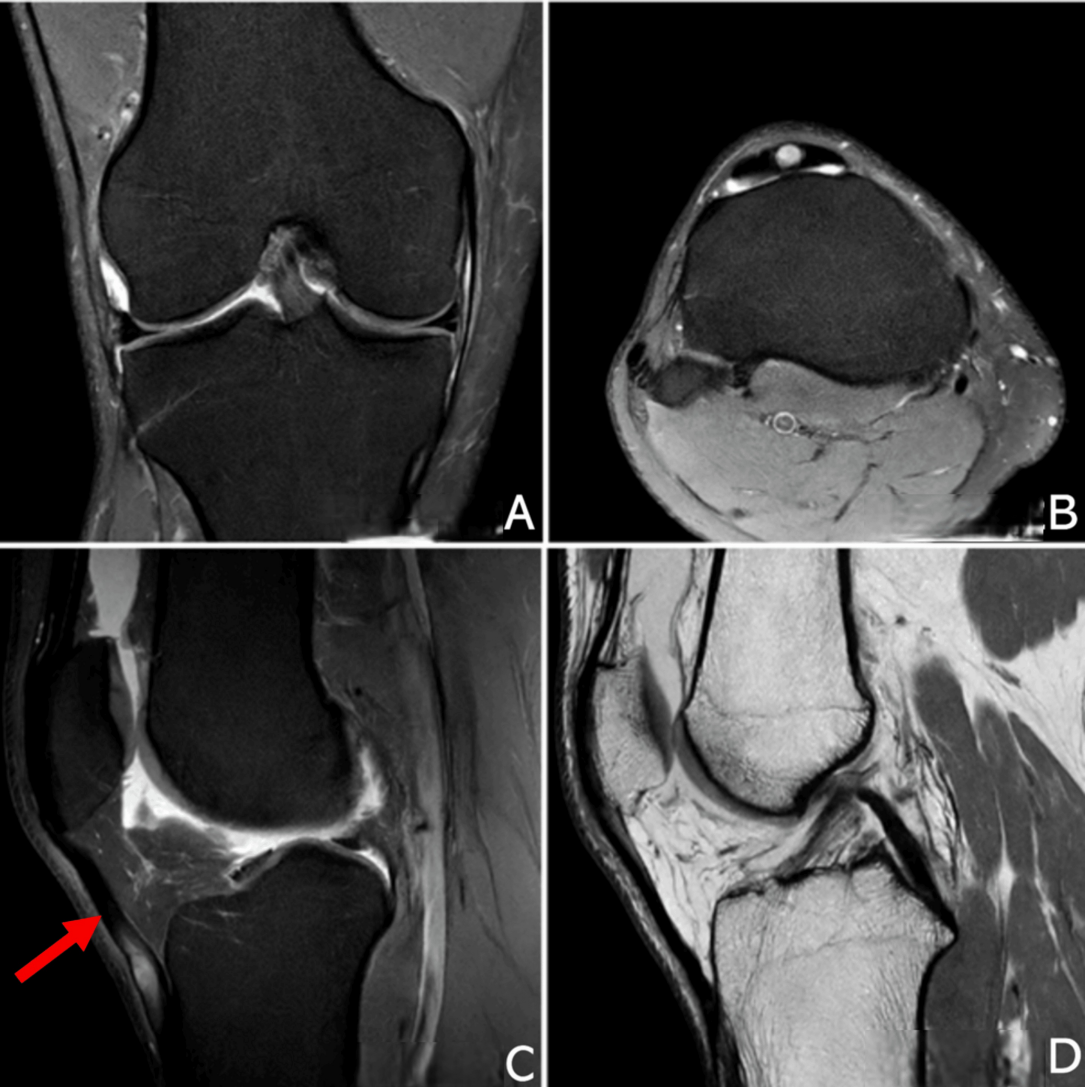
MRI T2-weighted images in the coronal (A), axial (B), and sagittal (C) views and T1 sagittal (D) view The image shows a patellar tendon intrasubstance tear with posteromedial meniscocapsular junction sprain without separation; intact anterior and posterior horns, body, and root attachments of the medial meniscus; and diffuse cartilage thinning on the weightbearing surfaces of the femoral and tibial condyles, without focal chondral ulcers, subchondral bony edema, osteochondral loose bodies, subchondral fracture line, and focal patellofemoral chondral ulceration/delamination. There was no evidence of lateral meniscal tear, and both cruciate ligaments are intact.
